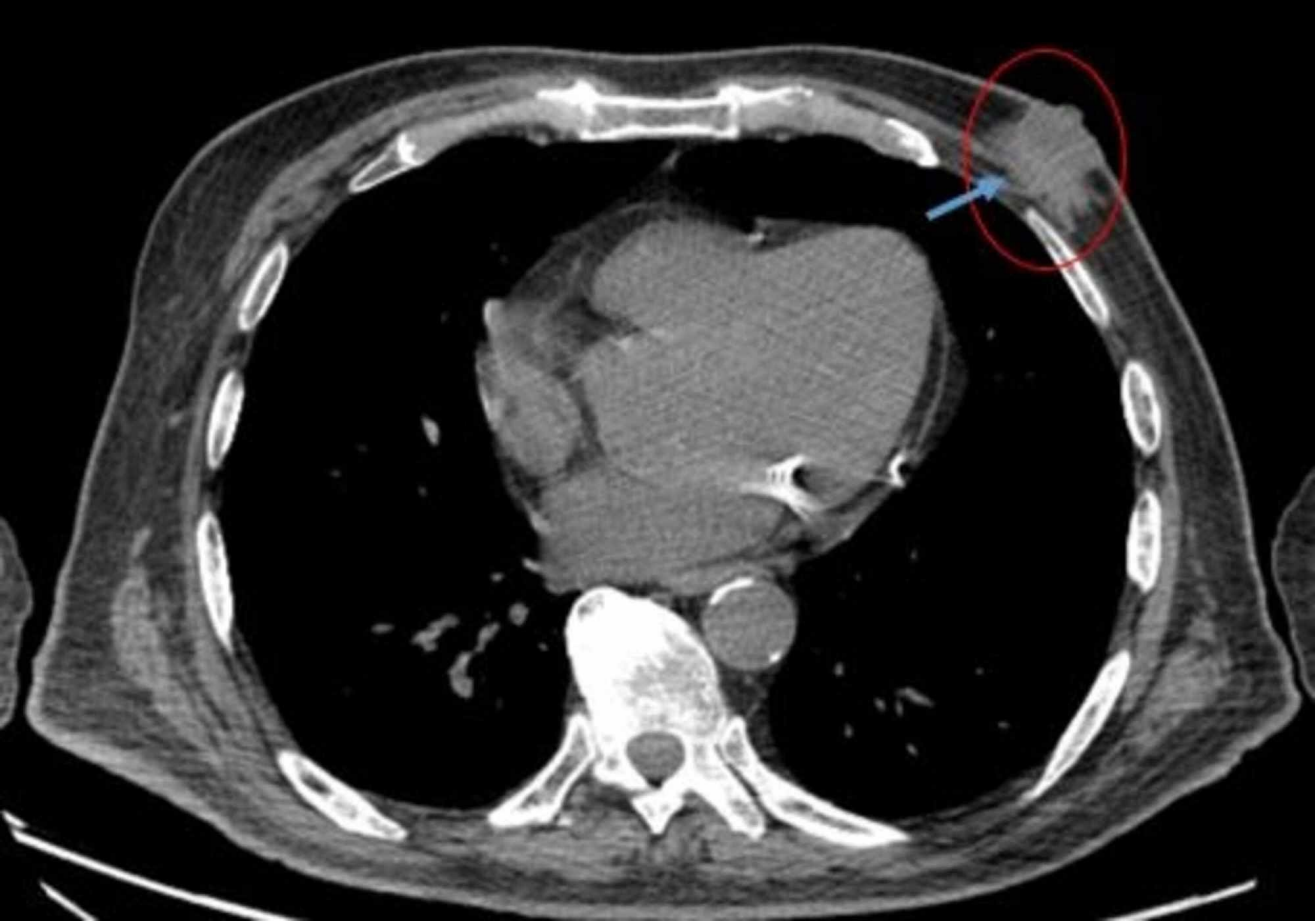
Chest CT of the patient There is a soft tissue density confluent with the left nipple measuring 3.2 cm identified on the image by the blue arrow and red circle. Dedicated breast imaging with mammography and ultrasound is recommended. Multiple subtle lytic lesions are seen in several ribs and left scapula, grossly unchanged and concerning for metastatic disease CT: computed tomography
